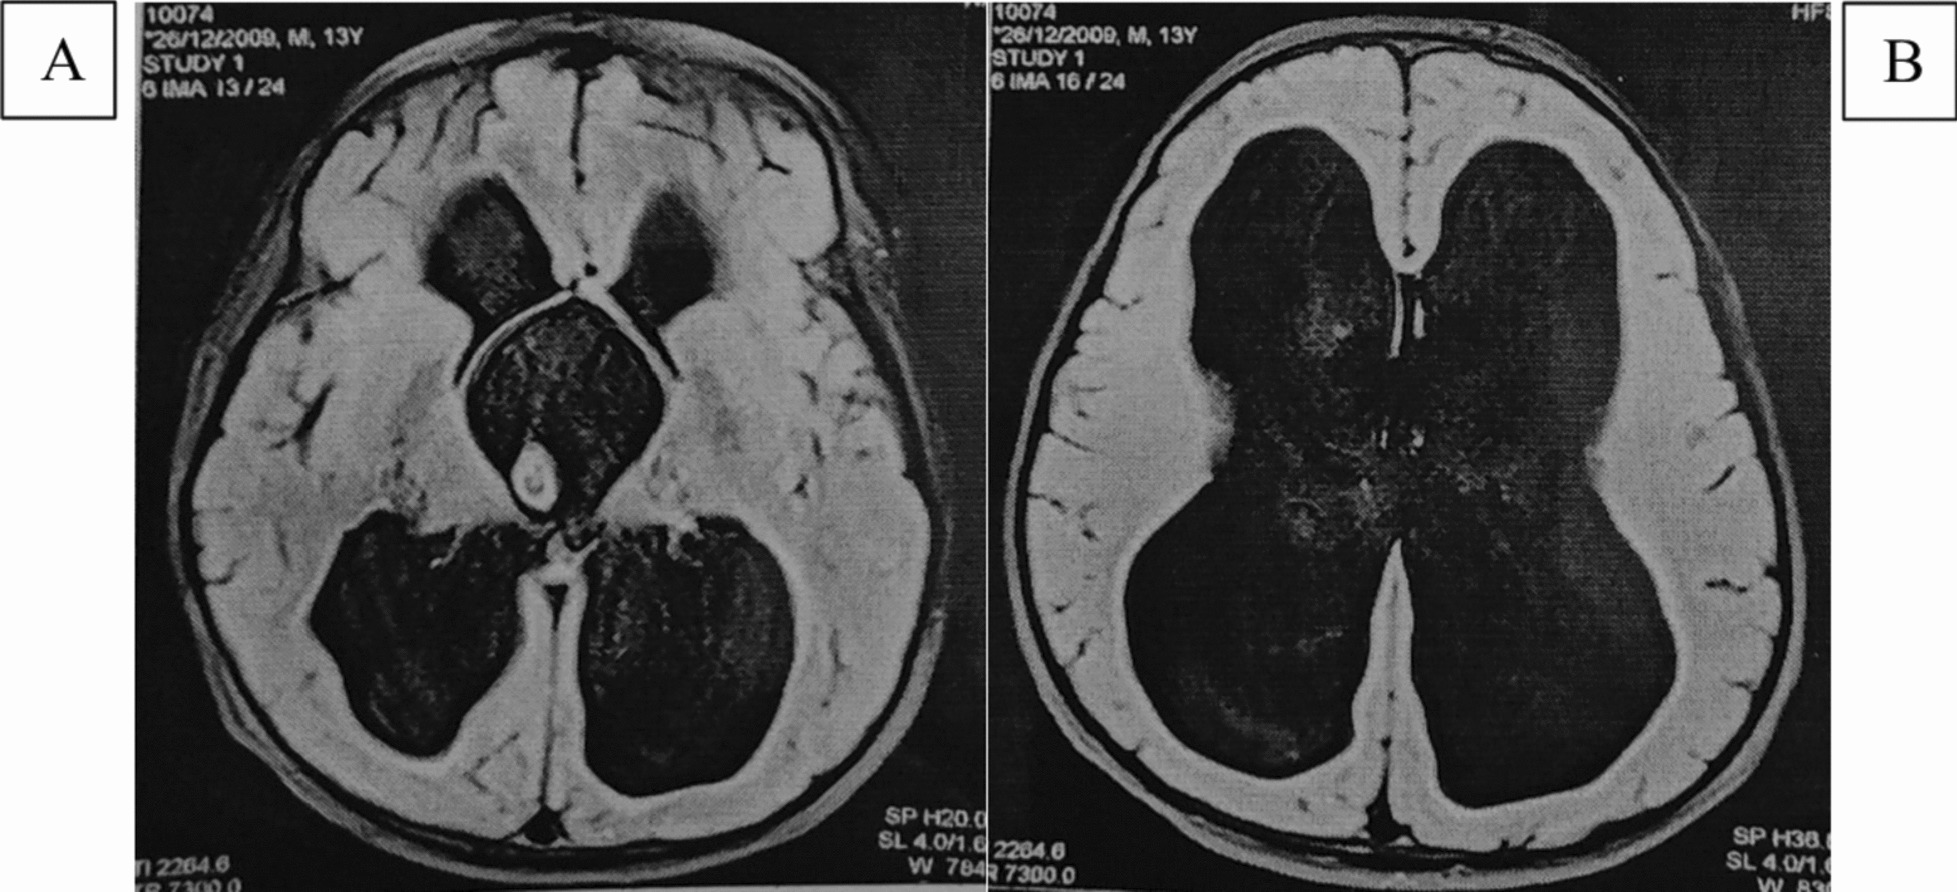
Axial FLAIR MRI of brain showing (A) dilated third and lateral ventricle, (B) dilated lateral ventricle
